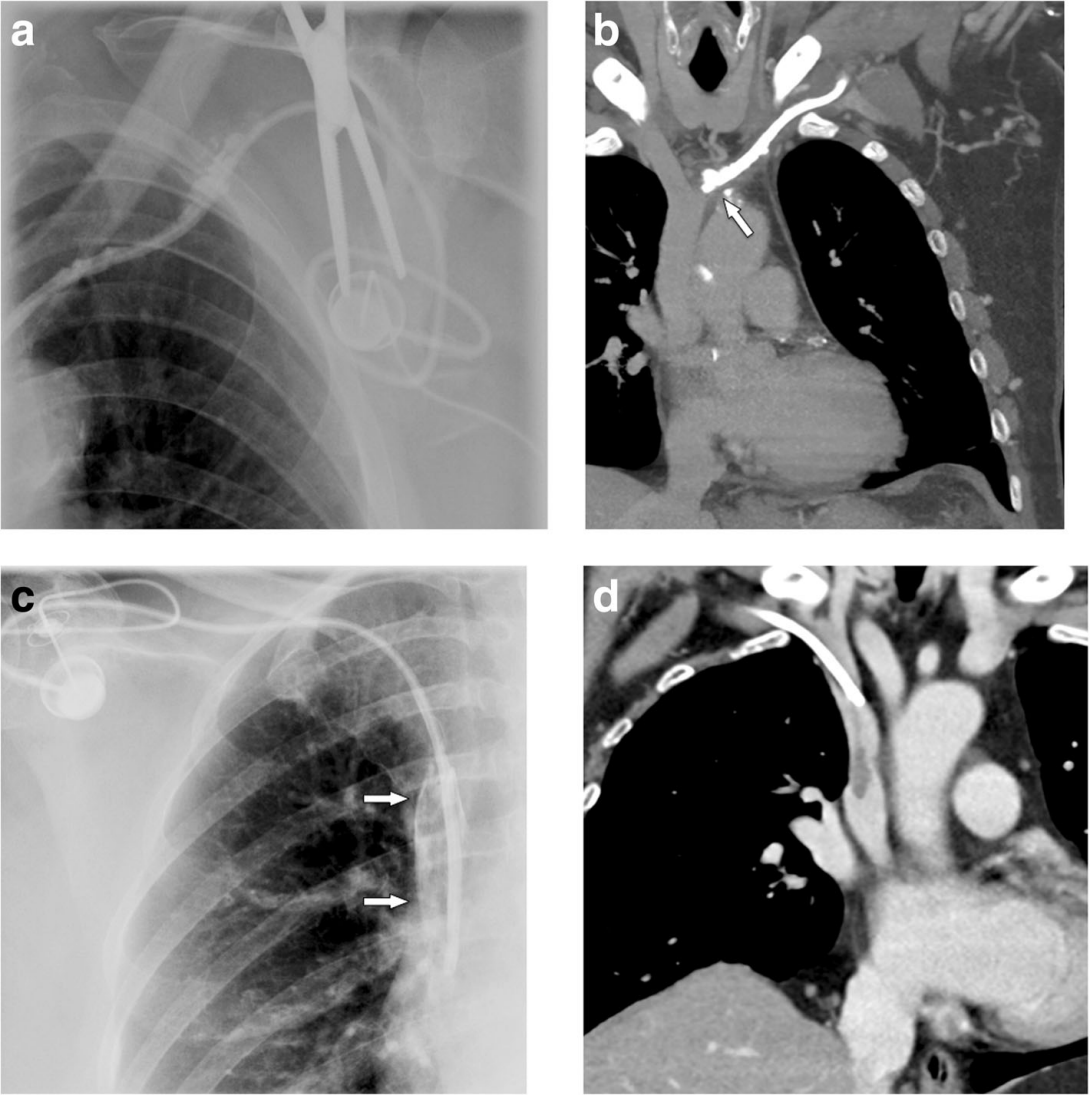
Thrombosis. a Flow confirmation study shows contrast backflow around the catheter. It is not clear, whether the catheter is in an intravascular position. b CT was subsequently performed, which shows the tip of the catheter too high in the brachiocephalic vein with venous thrombosis and development of multiple collaterals. c In another patient with increased resistance during infusions, flow confirmation study shows a fibrin sheath around the catheter (arrows). Infusion of thrombolytics (rTPA) restored good flow of the system. d In another patient, incidental finding of a thrombus at the catheter tip detected during contrast-enhanced MDCT follow-up
